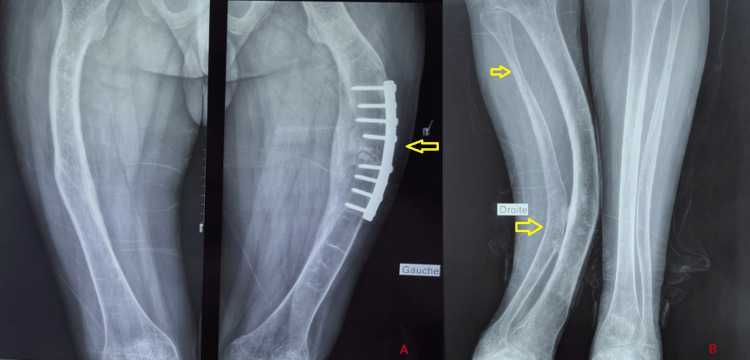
Anteroposterior radiograph of the lower limbs demonstrating: (A) Long-bone deformity with osteosynthesis hardware (arrow), associated with bowing. (B) Marked deformity of the long bones with cortical thinning (arrows), consistent with skeletal fragility suggestive of osteogenesis imperfecta.
